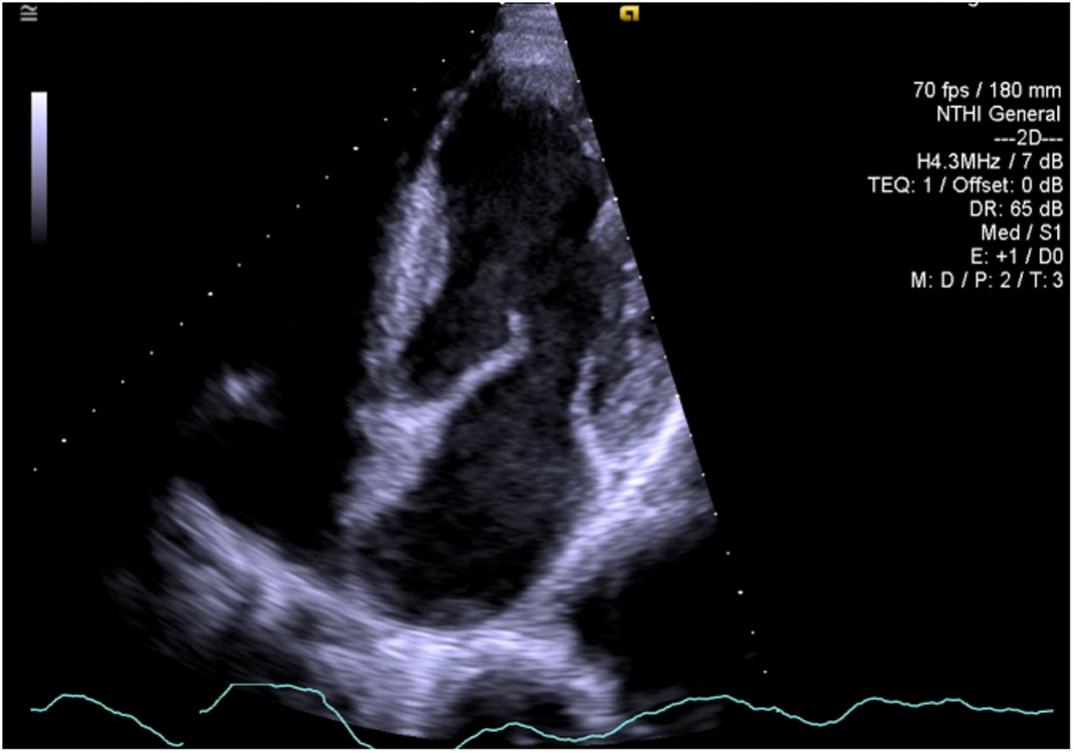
Left Ventricular Thrombus Resolution The transthoracic echocardiogram revealed complete resolution of the apical left ventricular thrombus.
